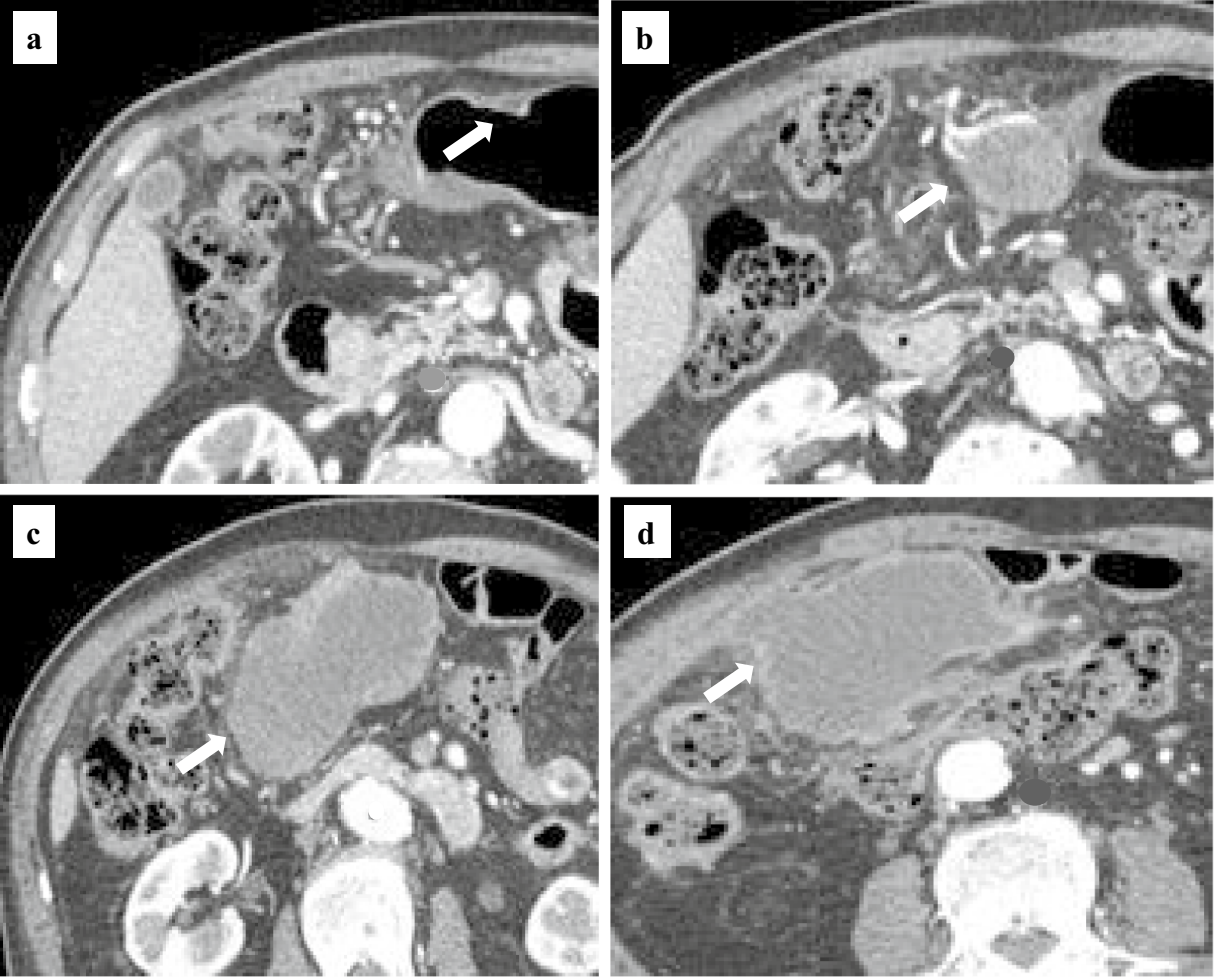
Abdominal enhanced CT: the 0-IIc lesion (a) and the giant tumor (b, c, d)
